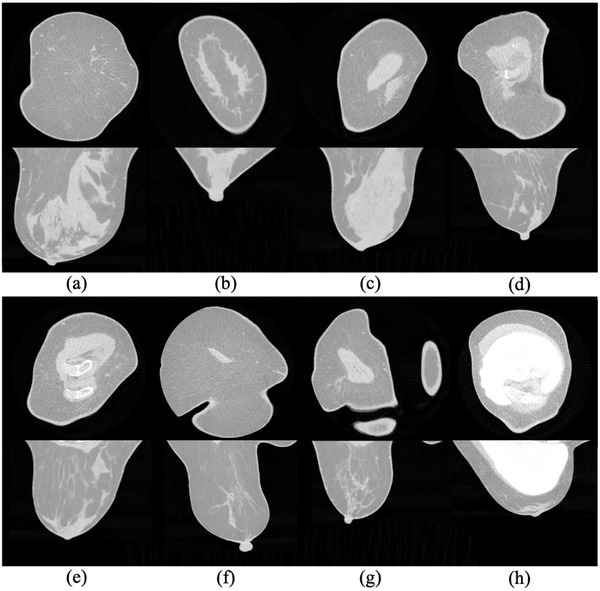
Eight exemplary spiral breast computed tomography (CT) images in coronal (top) and transverse or sagittal (bottom) planes
